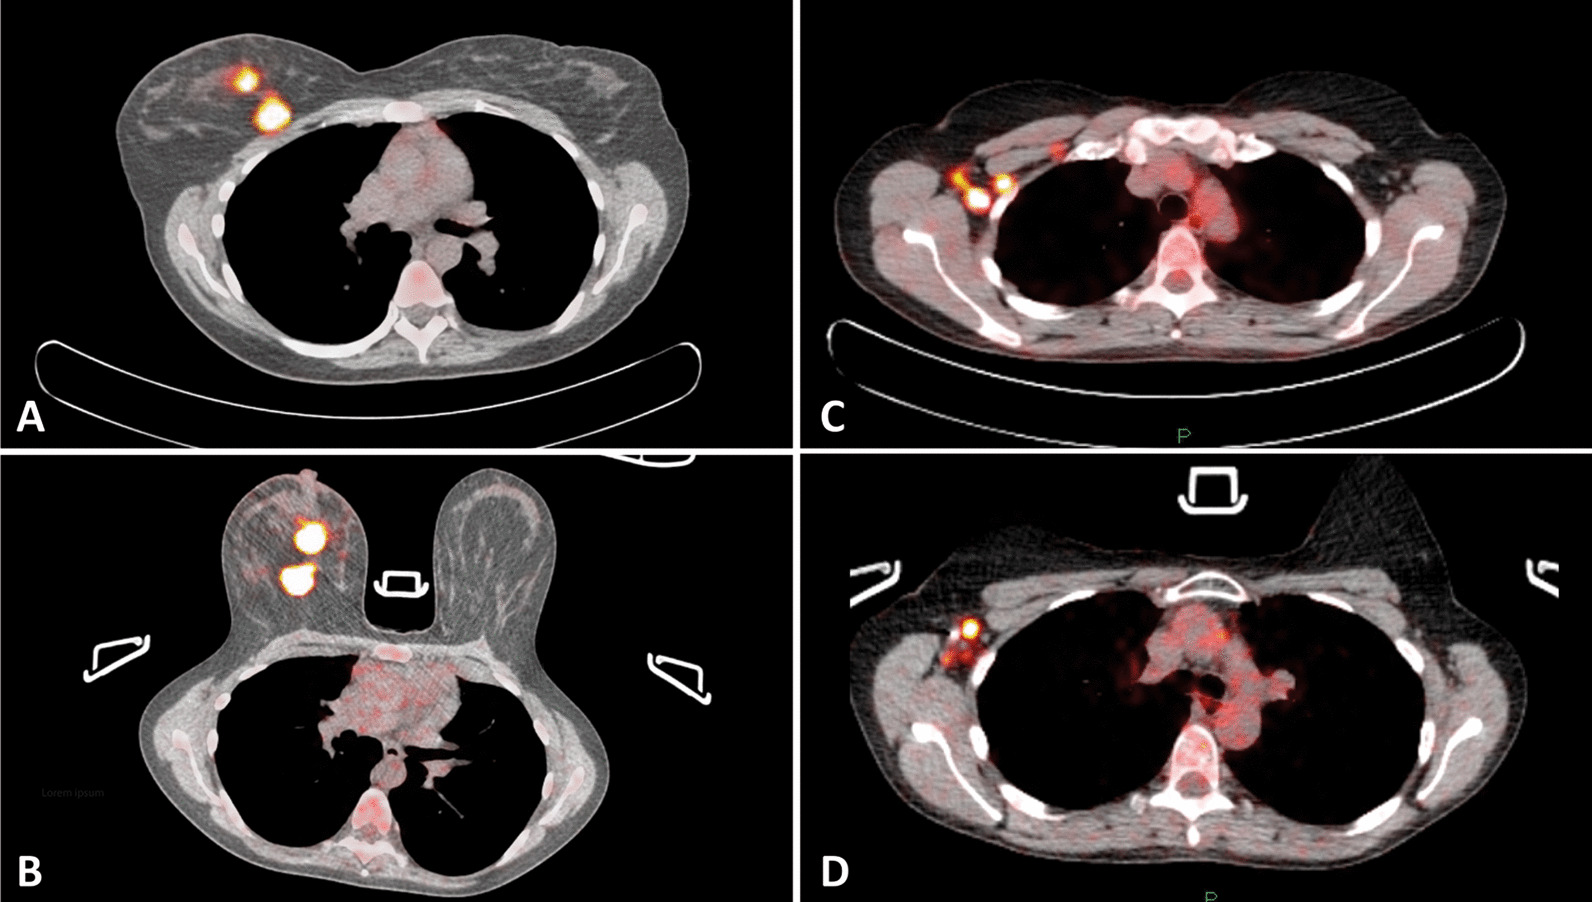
Patient with multifocal right-sided breast cancer with [^18^F]FDG-PET/CT performed in supine and prone position. Images A and C were acquired in supine position and images B and D were acquired in prone position, showing a multifocal FDG-avid primary breast cancer and multiple FDG-positive axillary lymph node metastases, respectively. In this patient, one additional FDG-positive ALN was counted in prone position compared to supine position
